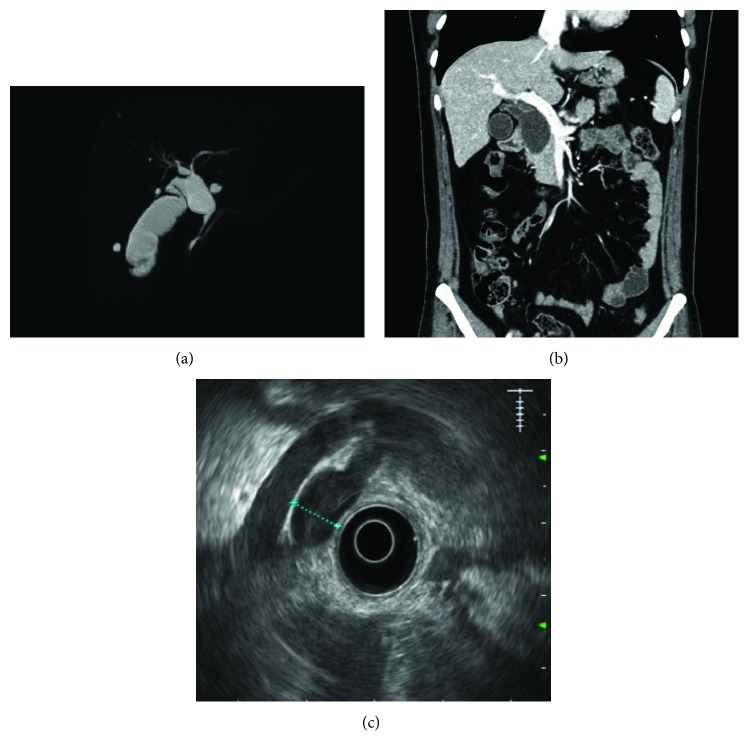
Characteristic findings of imaging modalities in patients with BDC. (a) MRCP reveals anomalous union of the pancreaticobiliary duct, and common bile duct dilatation involving both the left and right intrahepatic bile ducts. (b) CT scanning reveals cystic dilatation of the extrahepatic bile duct. (c) Endoscopic ultrasonography shows common bile duct dilatation. BDC: bile duct cyst; MRCP: magnetic resonance cholangiopancreatography; CT: computed tomography.
